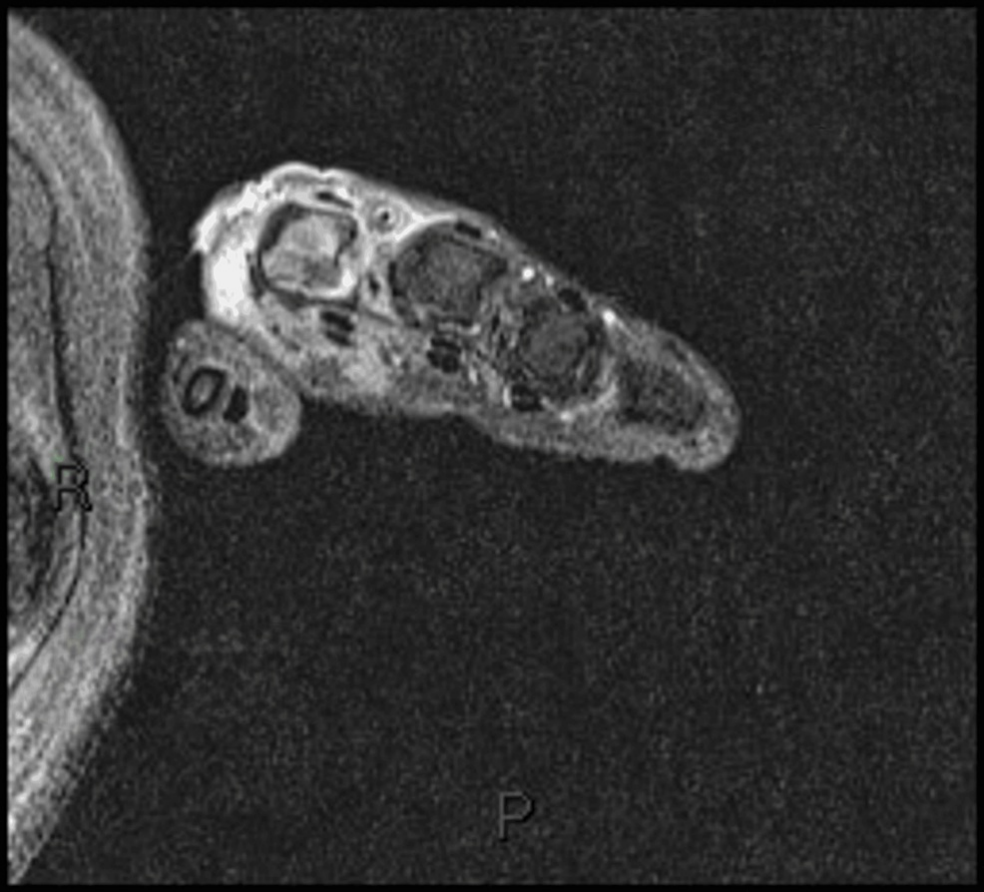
MRI of patient 1 showing inflammatory changes to the right MCP joint of the index finger with associated muscle damage MRI: Magnetic resonance imaging; MCP: Metacarpophalangeal.
